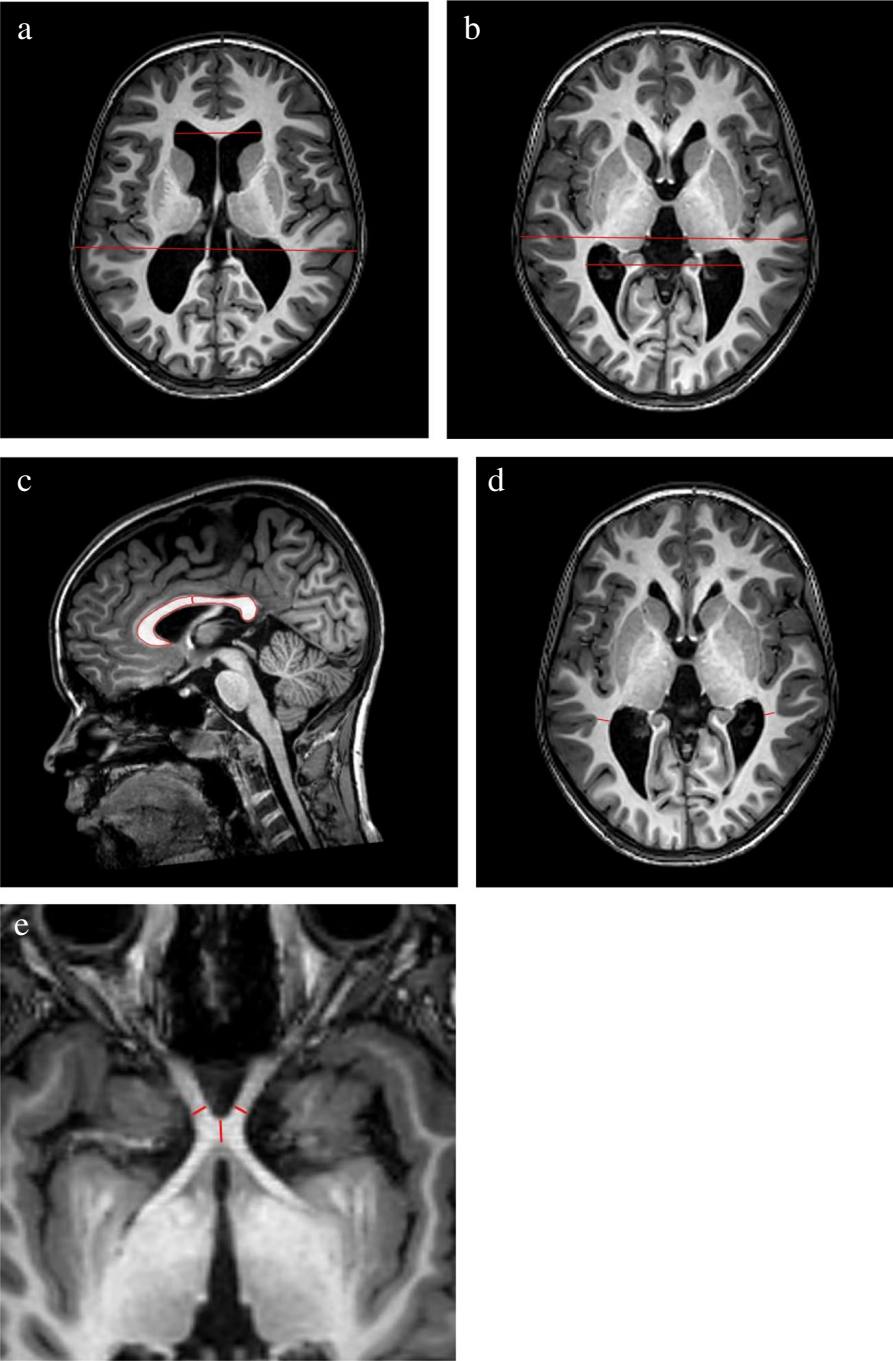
a The ratio of the maximum width of the frontal horns and maximal internal diameter of the skull at the same slice employed in axial MRI. b The maximum width of the posterior parts of the lateral ventricles (at the trigonum) were related to the maximal internal diameter of the skull. c The corpus callosum and the posterior part of the corpus callosum were assessed on a mid-sagittal slice. The posterior part of the corpus callosum was assessed by measuring the surface area of the posterior half. d The mean of the bilaterally smallest white matter part next to the trigone of the lateral ventricles was calculated in mm in order to assess the loss of white matter. e The thickness of the chiasma and the mean of the bilaterally optic nerve thickness was calculated in an axial T1-weighted slice
